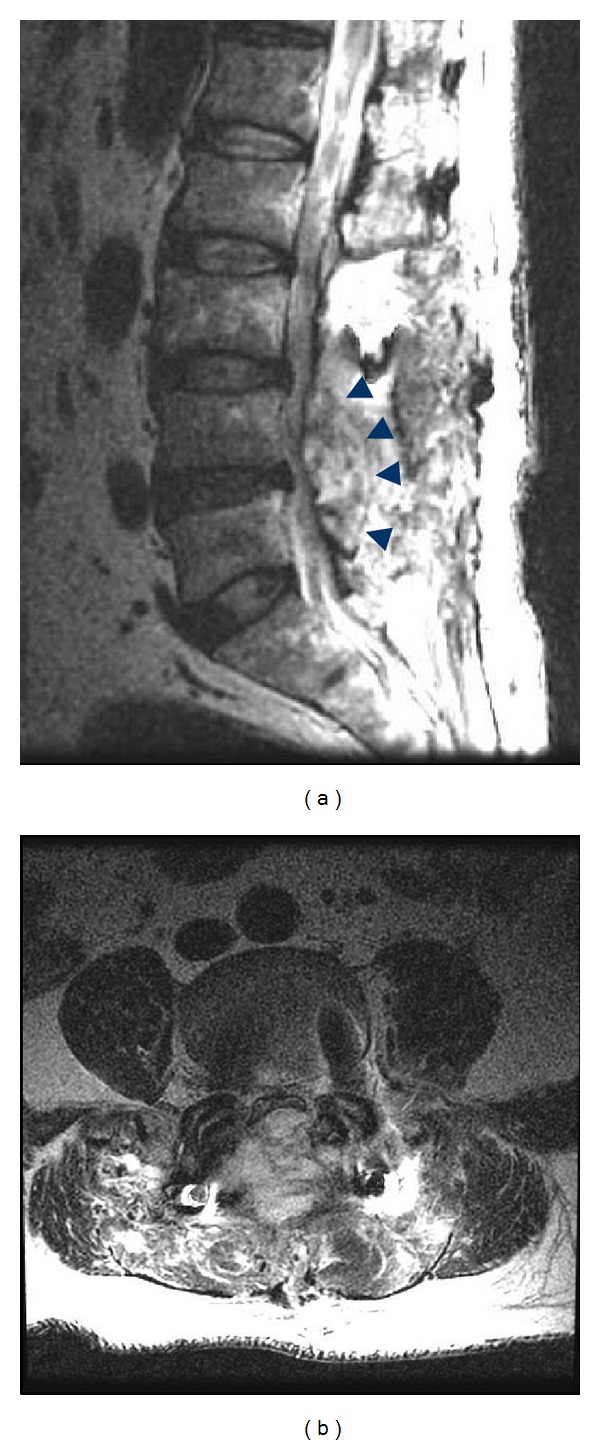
(a) Magnetic resonance imaging (MRI) T2-weighed sagittal image acquired 15 days postoperatively demonstrates a convex-shaped lesion at the surgical site (▲) compressing the thecal sac. (b) MRI T2-weighed axial image shows a posterior high intensity area completely flattening the thecal sac from both posterior right and posterior left sides.
